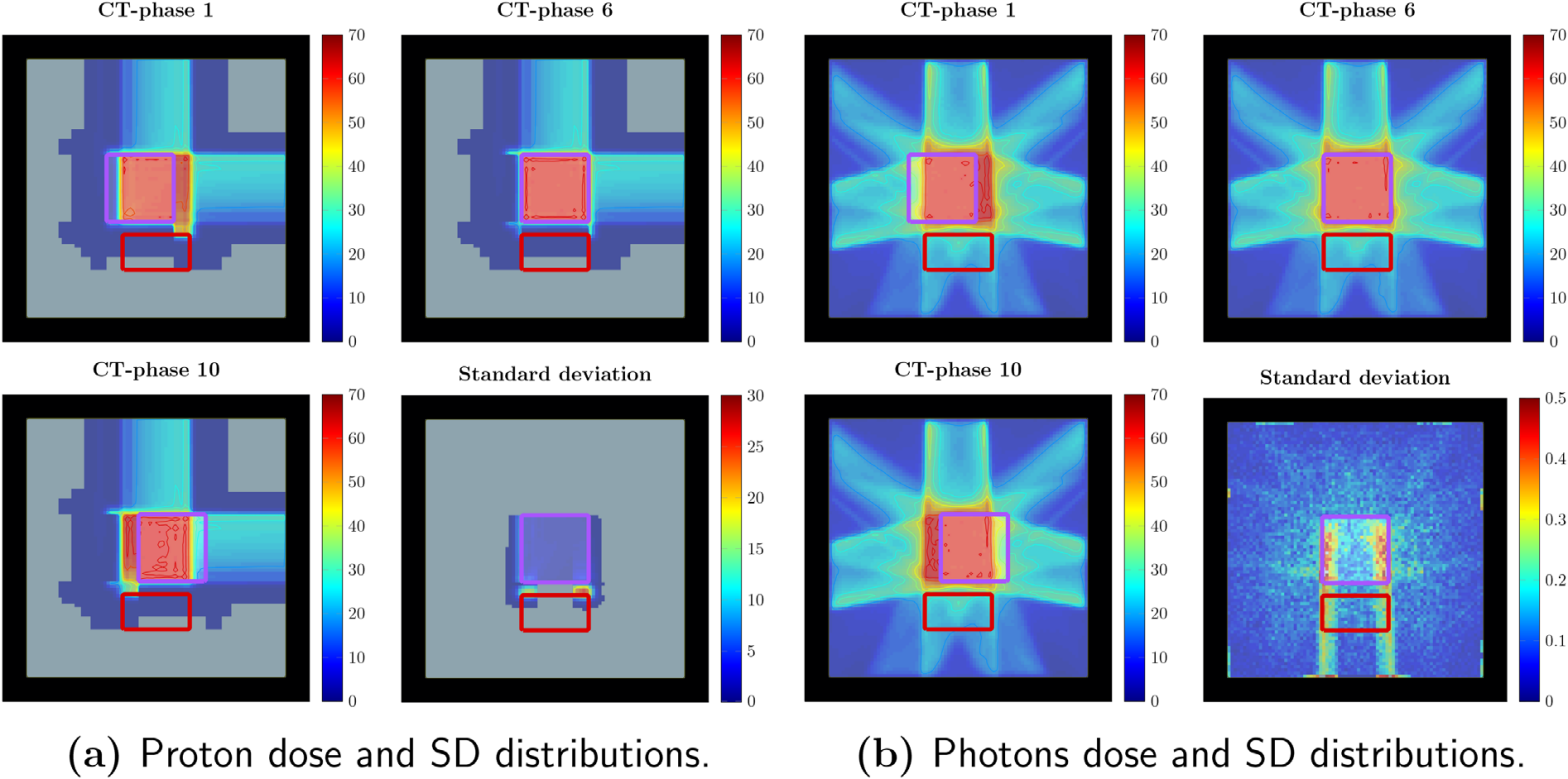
Dose distributions obtained for the CT‐phases at the lower (CT‐phase 1) and upper (CT‐phase 10) end of the motion range, nominal non‐deviated phase (CT‐phase 6) and the relative standard deviation distribution. The plans correspond to the proton (a) and photon (b) irradiation plans. The dose distributions are obtained as part of post‐optimization robustness analysis performed on the nominally optimized distribution. The contoured structures correspond to the Target structure (purple) and the distal organ at risk (red). All colorbar values are reported in Gy.
